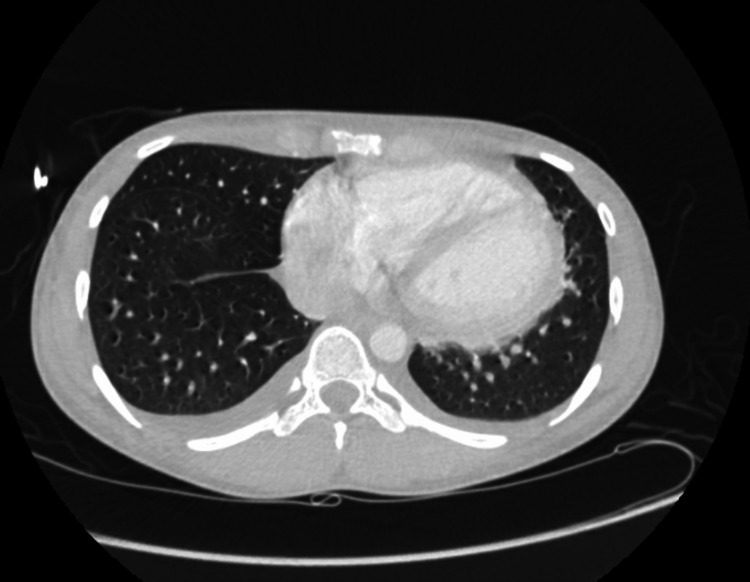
CT chest (transverse) showing cardiomegaly and some signs of fluid overload
